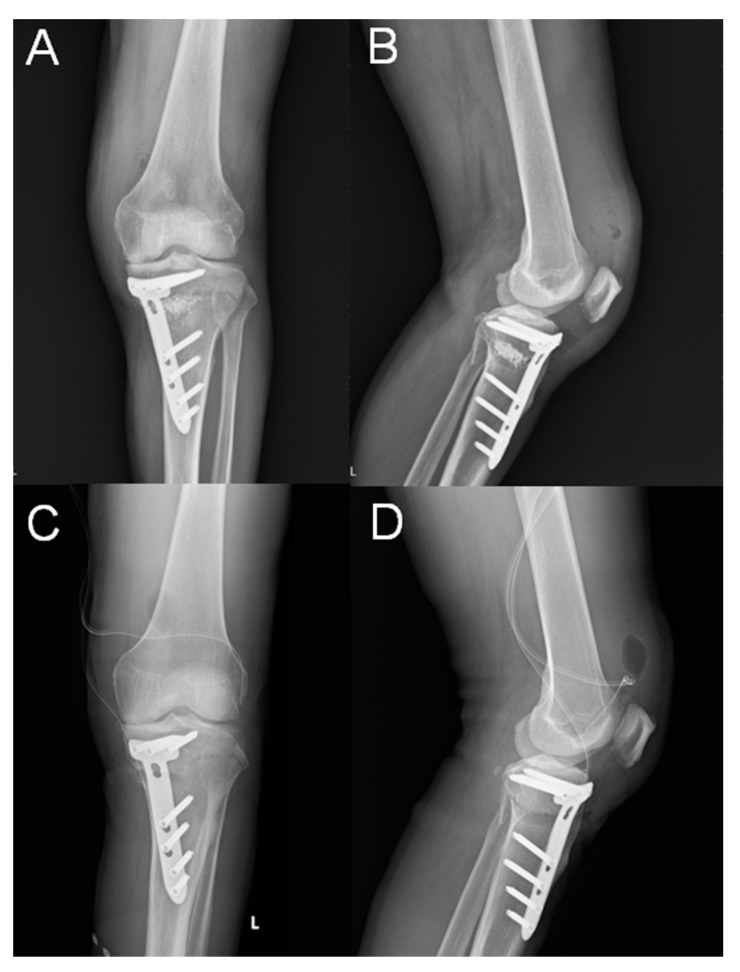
(A,B) A 58-year-old male presented with an acute deep SSI 2 weeks after MOWHTO. Plain radiographs with anteroposterior and lateral views showed that the Tomofix locking plate position was slightly more anterior than standard position, and the artificial bone graft could be seen at the osteotomy site. (C,D) After debridement surgery, the artificial bone graft was removed, and a drainage tube was placed in the knee joint and at the osteotomy site.
